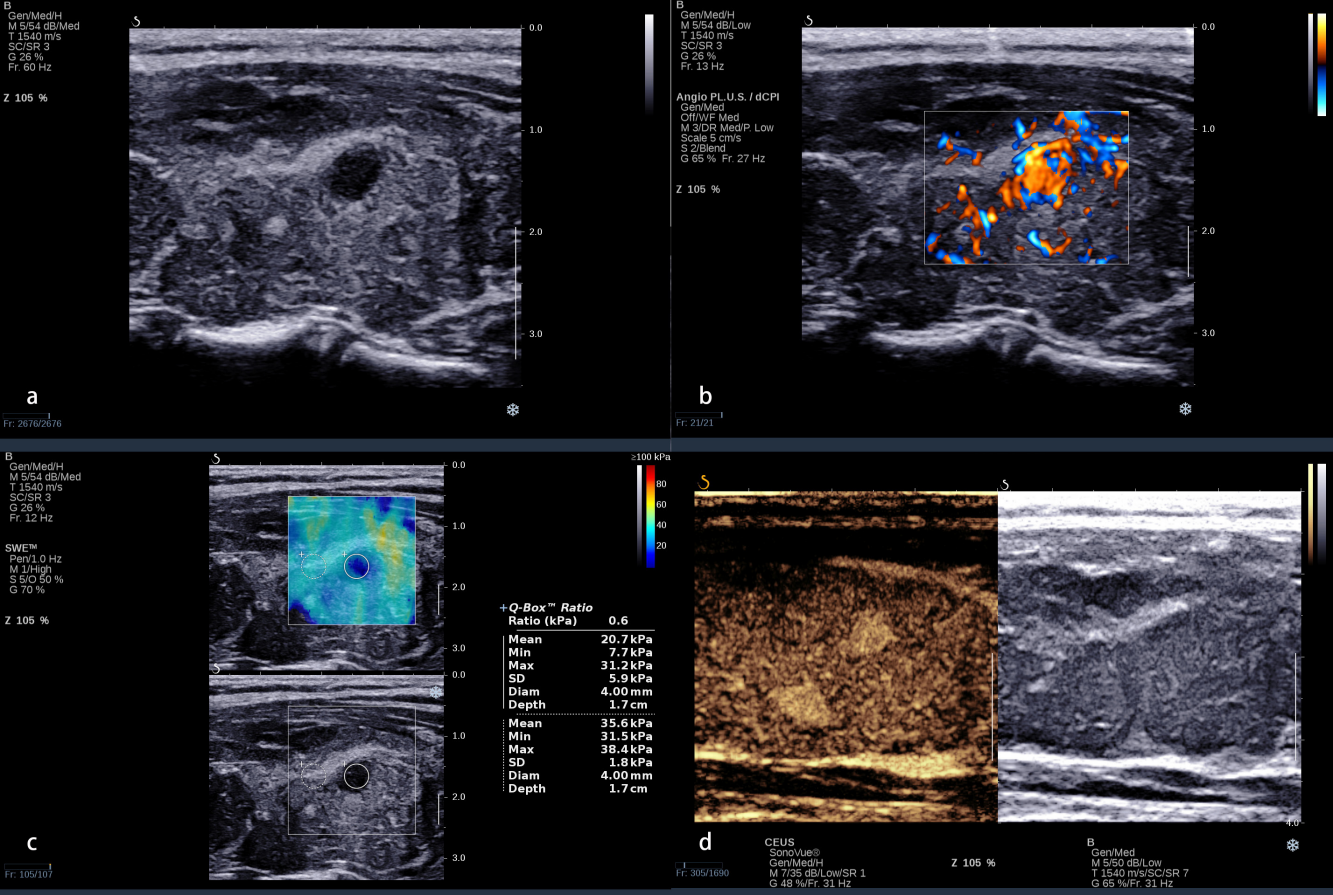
A 32-year-old woman with a nodule in the left lobe of the thyroid. (A) Conventional ultrasound revealed a 5 × 6-mm solid hypo-echoic nodule with a smooth margin, shape that was taller-than-wide, and without obvious calcification; this was categorized ACR TI-RADS 5. (B) The nodule presented a rich blood flow signal. (C) Quantitative shear wave elastography (SWE) revealed 7.7 kPa for Emin, 20.7 KPa for Emean, 31.2 kPa for Emax, and 0.6 for Eratio. (D) Qualitative contrast-enhanced ultrasound (CEUS) of the nodule revealed homogenous, hyper-enhancement. The combination of CEUS and SWE revealed that this nodule may be benign. The surgical pathology was focal Hashimoto’s thyroiditis.
